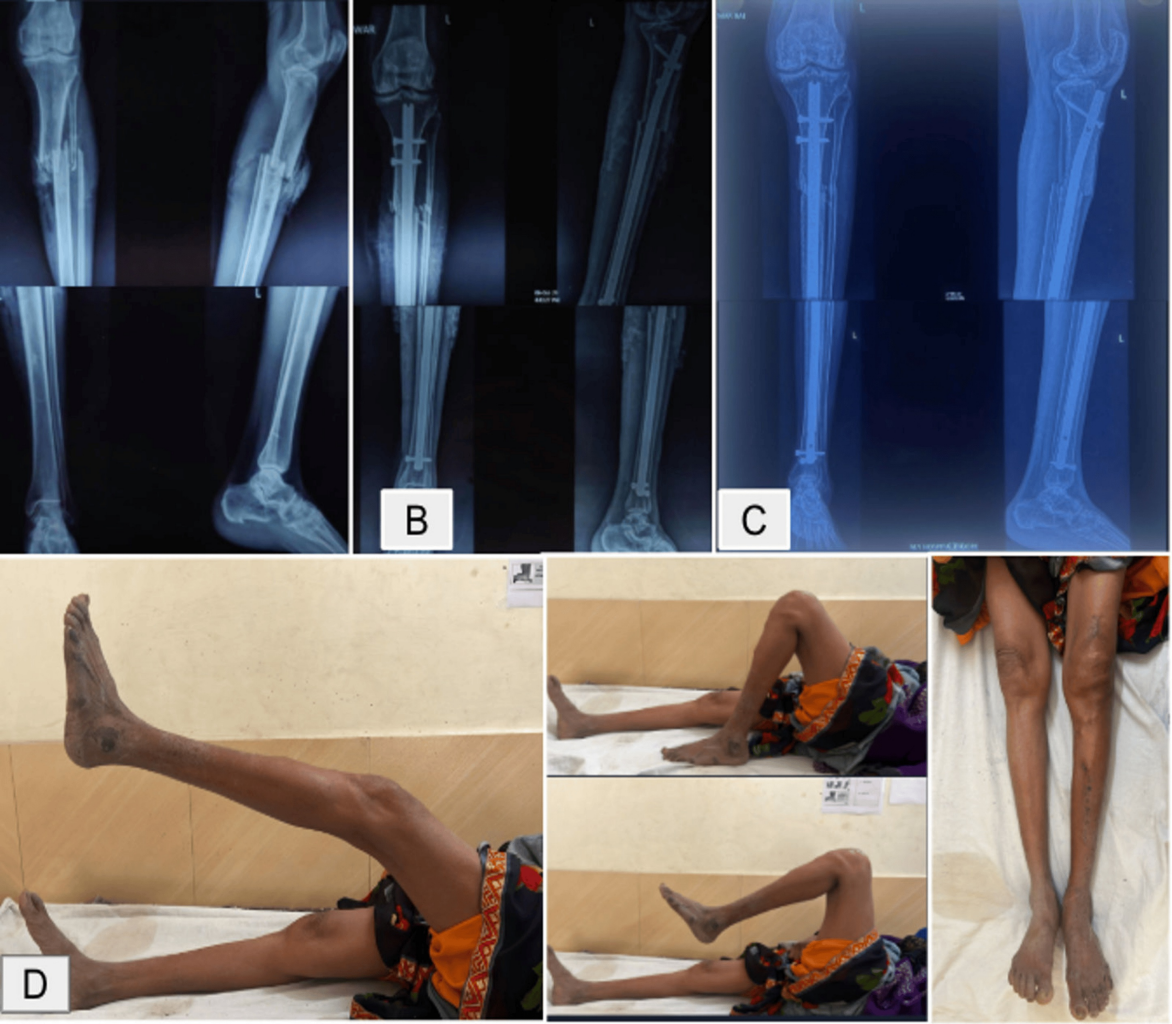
Case 2 of suprapatellar nailing. (A) Preoperative X-ray. (B) Immediate postoperative X-rays. (C) Three months postoperative X-rays. (D) Clinical assessment and knee range of motion at three months.
